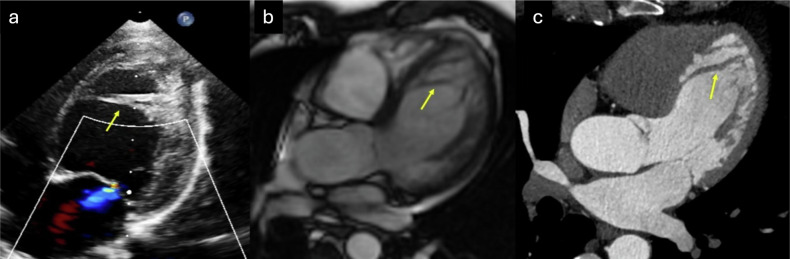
False tendons (aberrant bands) in two different patients studied with US, CMR, and cardiac CT, ((a) US in a newborn shows a dilated LV with a large aberrant muscular band (arrow) connecting the interventricular septum to the lateral free wall, which may be mistaken for ET, (b) SSFP cine CMR three-chamber view, and (c) cardiac CT) depict large aberrant muscular bands (arrows) mimicking apical ET. Both CMR and cardiac CT, through multiplanar evaluation and high contrast resolution, allow accurate definition of the anatomy and course of the aberrant bands
